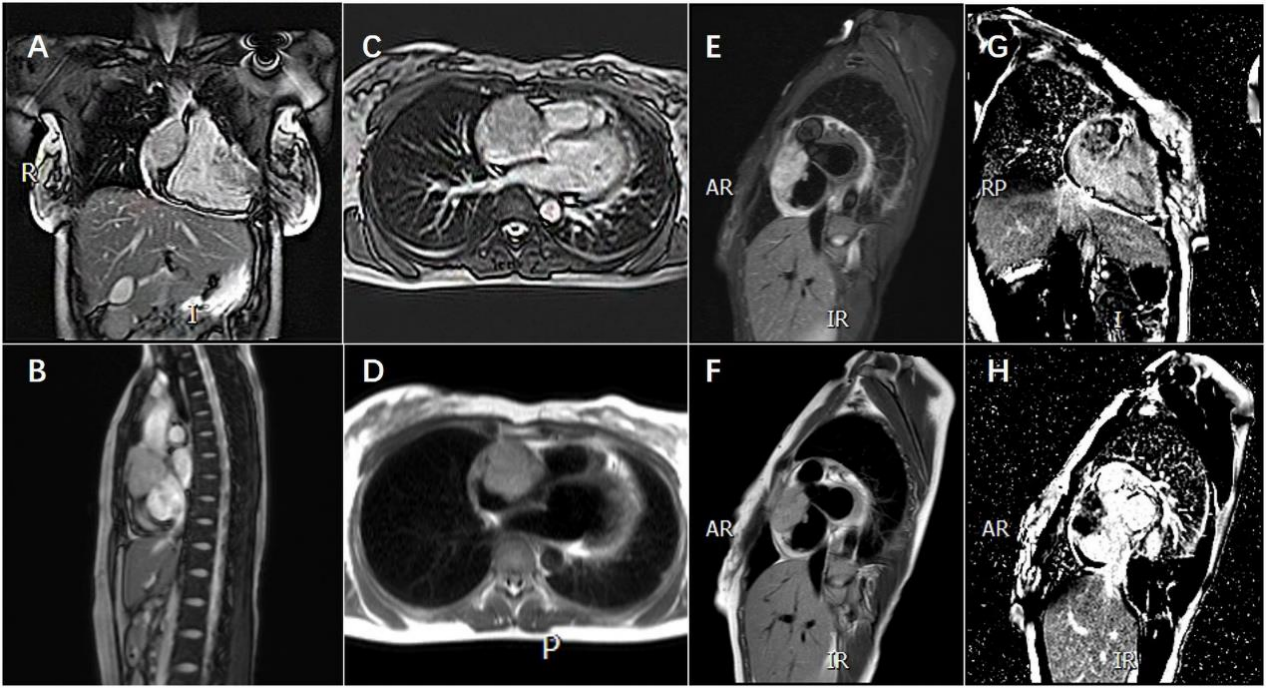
Different images of cardiac angiosarcoma during cardiac magnetic resonance imaging. (A-C) On bright-blood T2-weighted MRI, there was an iso intensity soft tissue mass. (D, F) On dark-blood T2-weighted MRI, four-chamber and short-axial view confirmed infiltration of the right atrium free wall and atrioventricular groove. (E) The mass showed hyperintense on the T2-weighted turbo inversion recovery magnitude (T2w-TIRM) sequence. (G, H) Short-axial late gadolinium enhancement (LGE) on CMR appeared to be a heterogeneous pattern of delayed enhancement.
